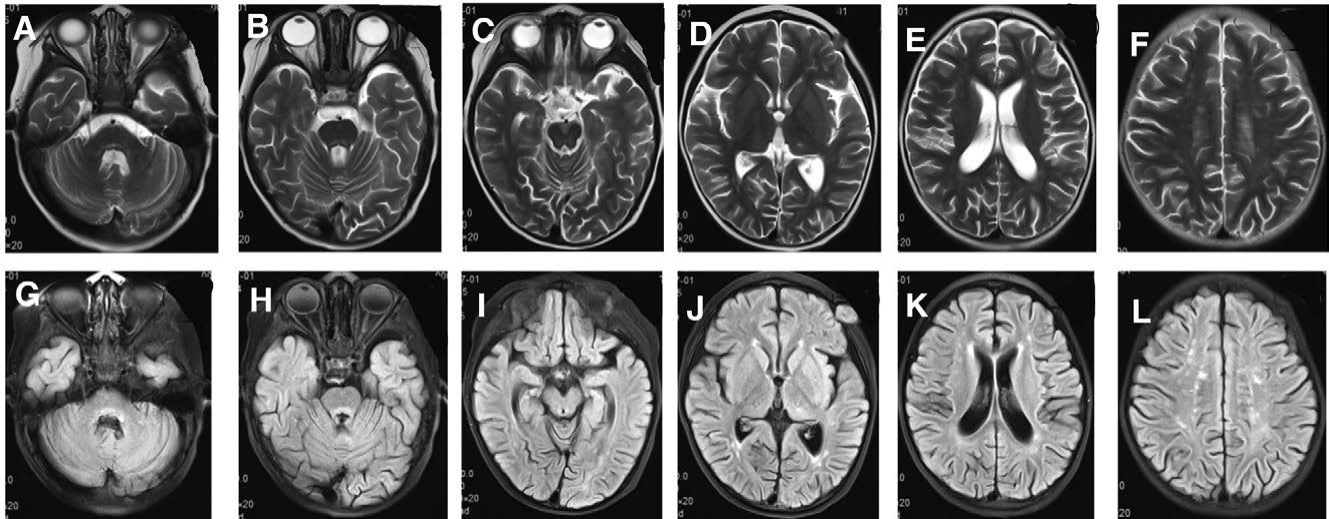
Cranial MRI of Case 1 at the age of 3 years and 4 months. Multiple patchy, slightly long T1, long T2, and FLAIR high signals found at white matters of bilateral frontal, parietal, and occipital lobes, as well as bilateral para-ventricular white matters, left thalamus, bilateral cerebellar hemispheres, and dorsal pons, with relatively clear boundaries. Some lesions had a small round shape. The lesions at bilateral cerebellar hemispheres, left thalamus, and dorsal pons was slightly smaller than before, and the FLAIR signals were reduced. However, the lesions of white matters of bilateral parietal and occipital lobes increased. The posterior parts of the bodies of bilateral lateral ventricles are slightly widened. FLAIR = fluid-attenuated inversion recovery, MRI = magnetic resonance imaging.
